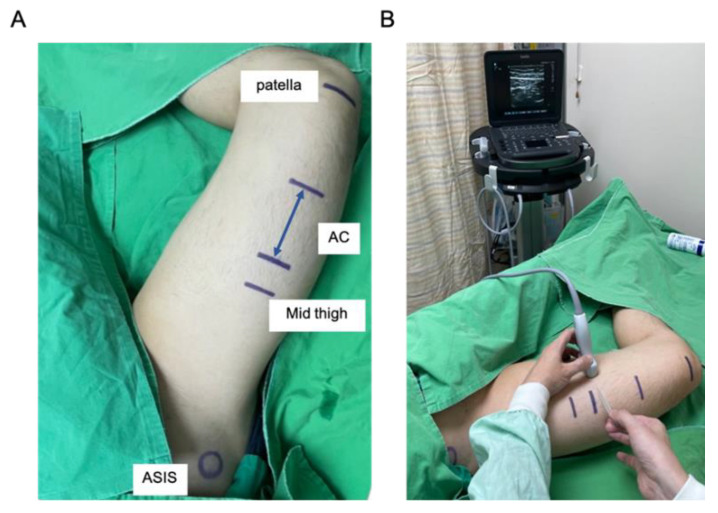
Ergonomic position for proximal adductor canal block. (A) Patient position for mid-thigh localization. The patient is in a supine position with the hip externally rotated and the knee slightly flexed. (B) Operator and ultrasound machine position. Operators stand same side and lateral to the leg, hold the needle with dominant hand, and position the ultrasound machine on the opposite side of the patient. AC, adductor canal; ASIS, anterior superior iliac spine.
